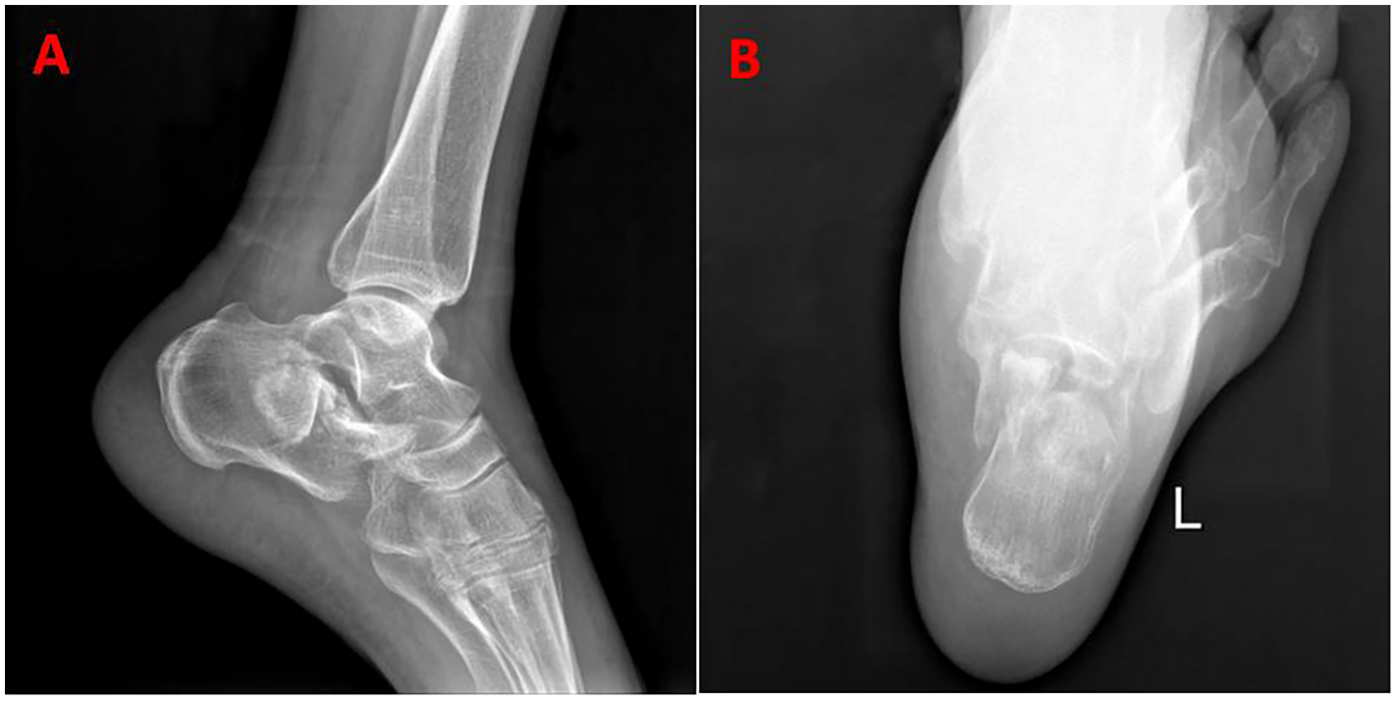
(A,B) Preoperative x-ray of the left foot shows a calcaneal fracture.
